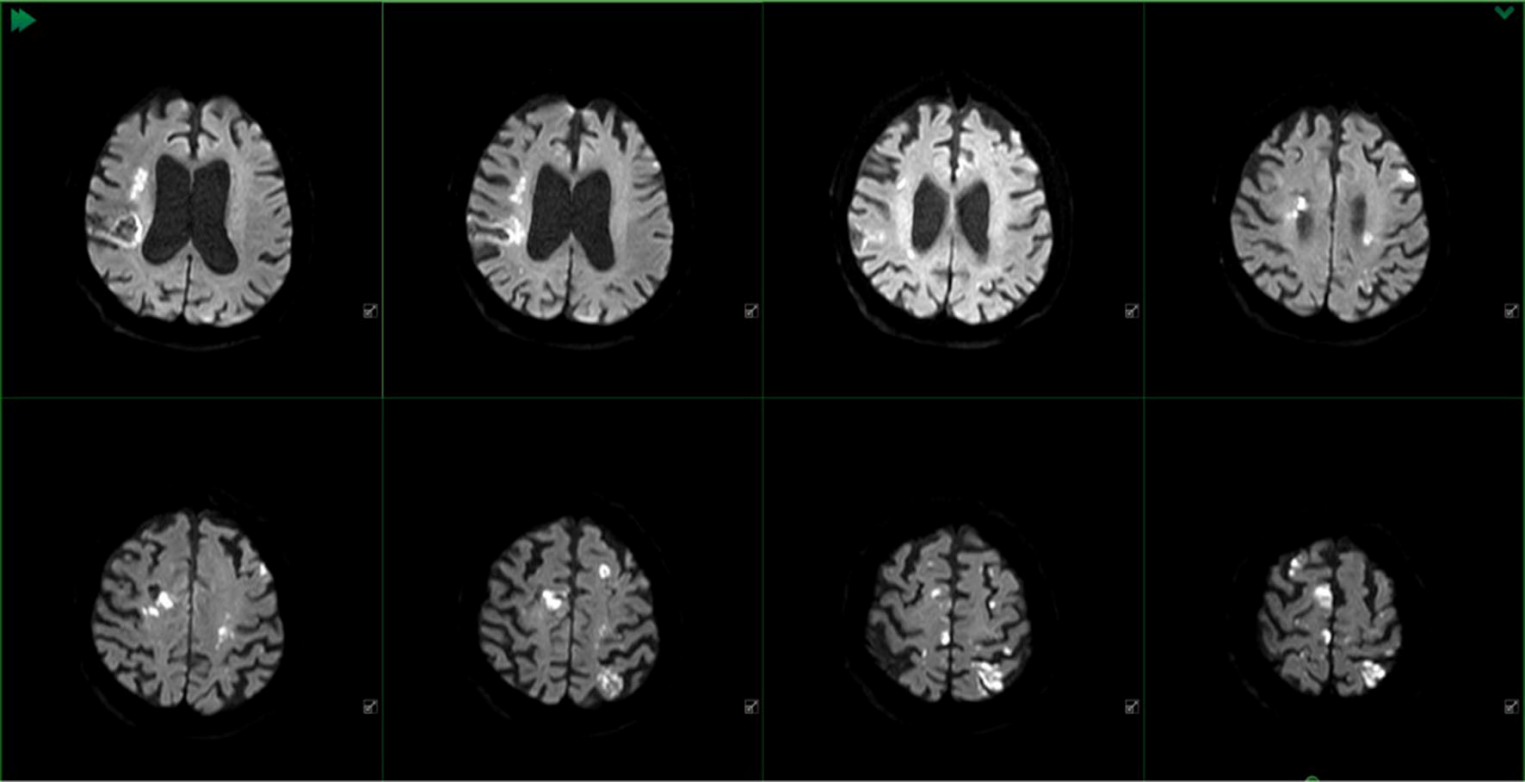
MR DWI of the patient before surgery with documentation of hemodynamic ischemia districts in both hemispheres.
